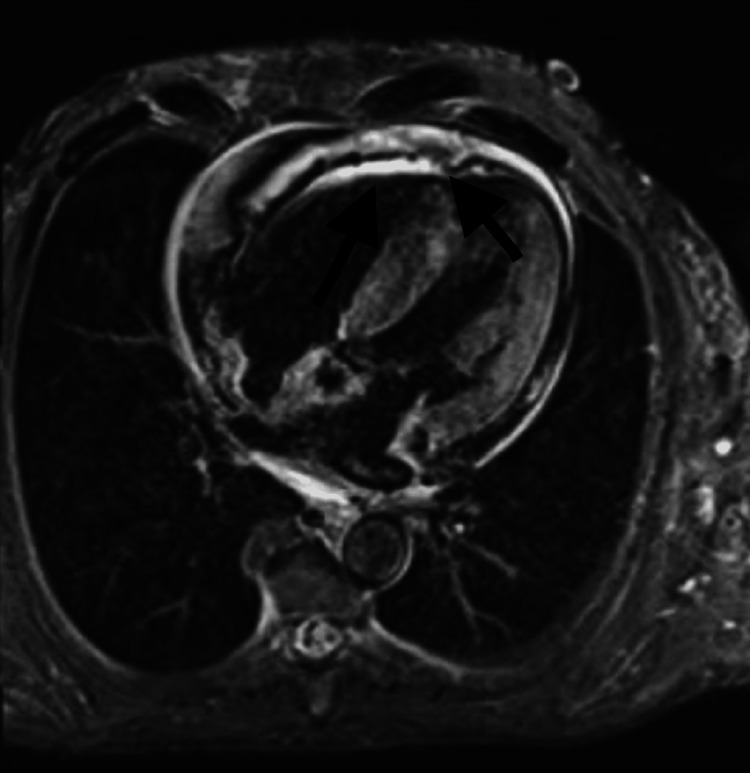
cMRI showing diffuse LGE consistent with amyloid (black arrows) cMRI: cardiac magnetic resonance imaging (cMRI); LGE: late-gadolinium enhancement
